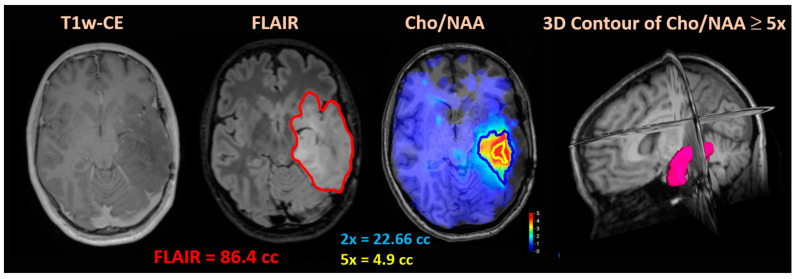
Patient with low-grade glioma presented for repeat biopsy to assess possible disease progression. Due to lack of enhancement in CE-T1w and difficulty finding the areas most suspicious for a progressive tumor within the FLAIR volume, EPSI was utilized to better delineate the target for biopsy. As noted, the FLAIR volume (red) of 86.4 cc is too large to allow for adequate targeting during surgical biopsies. A Cho/NAA ratio five times (yellow) abnormal was instead utilized to delineate the biopsy target. The 3D contours of Cho/NAA ≥ 5x (yellow) and Cho/NAA ≥ 2x (blue) are shown for a comparison. The 2x volume is typically used for radiation treatment, while the 5x threshold maximizes tumor density and probability of a successful biopsy. All images are displayed in our in-house program, the Brain Imaging Collaboration Suite (BrICS).
